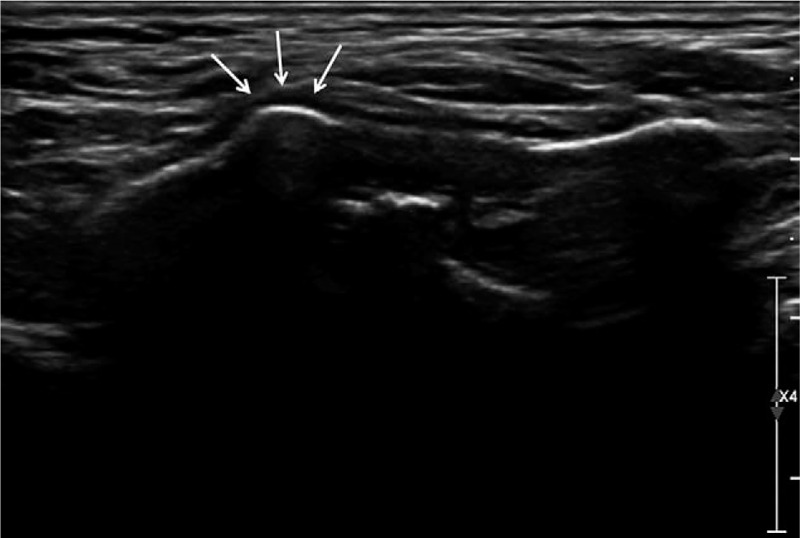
Ultrasonographic finding of right ossified stylohyoid ligament and identified tender point (arrows).
